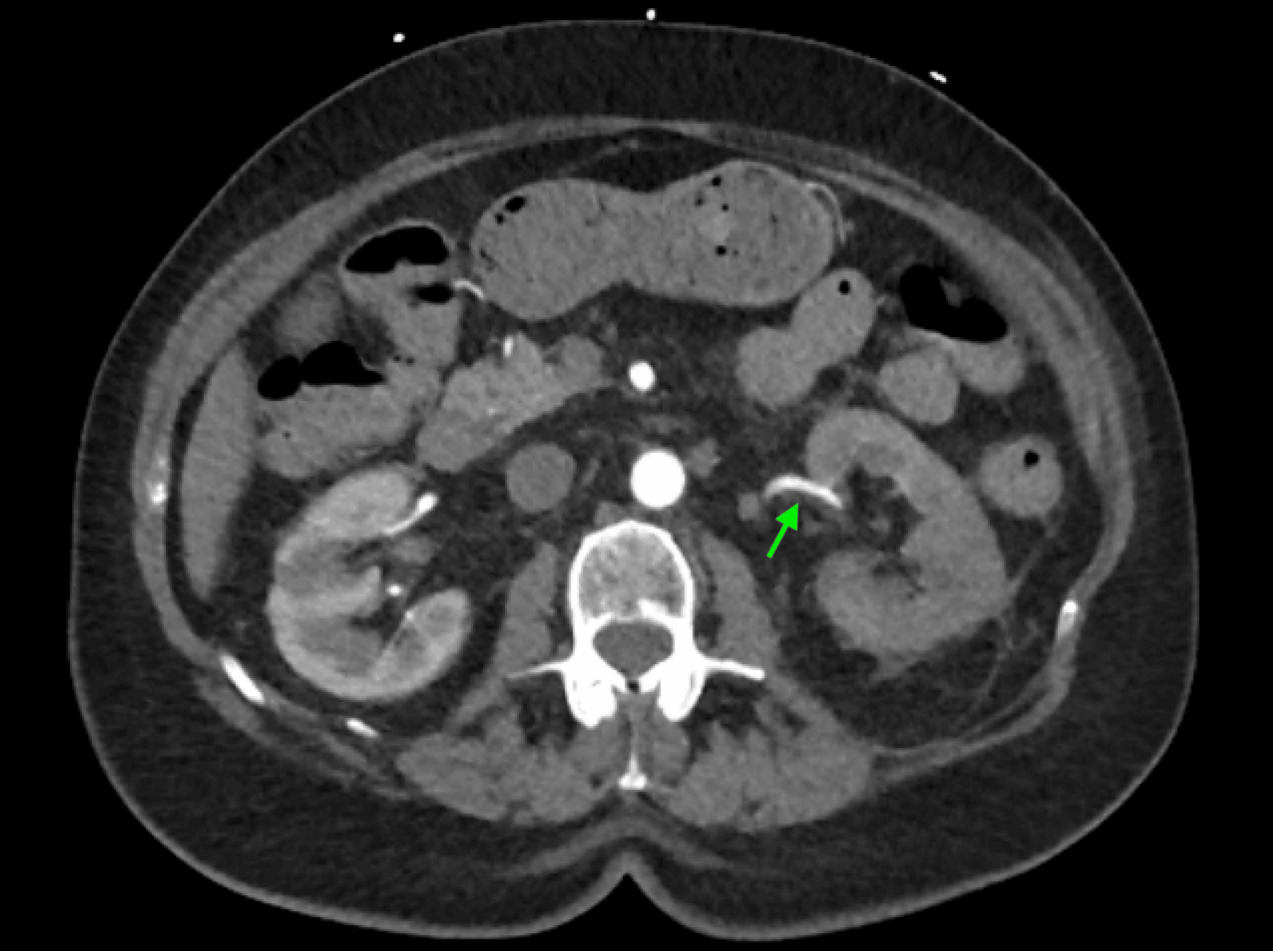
Axial CT angiogram of the whole aorta The green arrow points to the left renal artery, which now shows normal contrast opacification, indicating resolution of a previously present thrombus.
